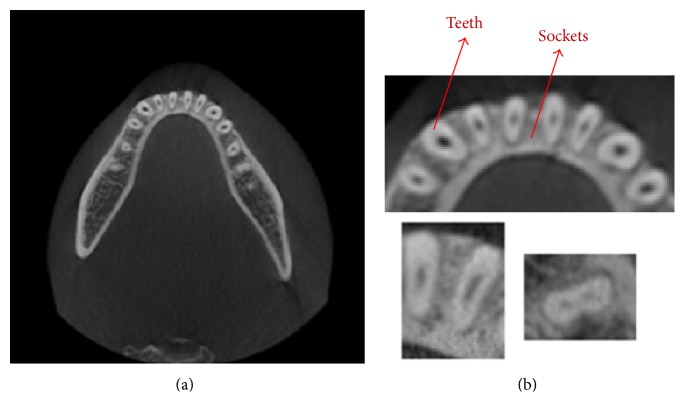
The axial view of the human mandible which is extracted with a threshold value: (a) is the original image of CT and (b) is the magnified view of the original image.
